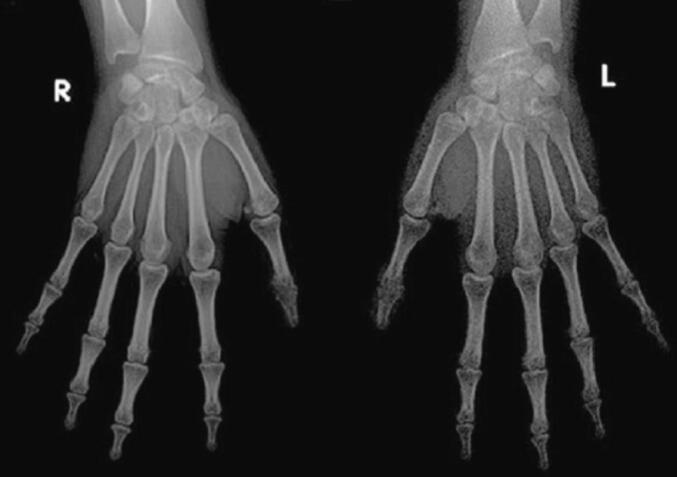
X-ray of both thumbs at six months post-operative condition.
